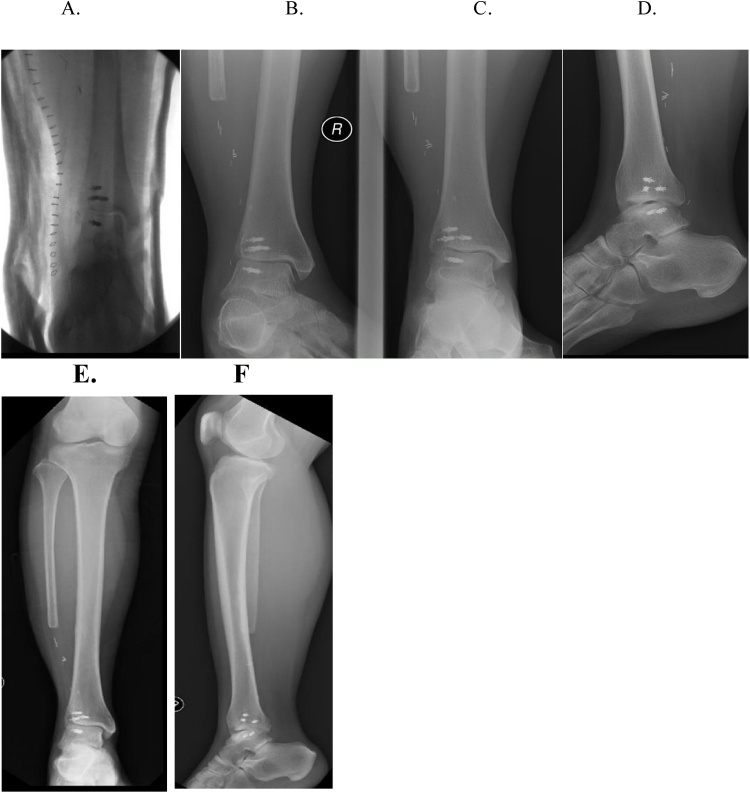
A) Immediate postoperative radiographs of patient #1 A, B, C, D) Two years post-op. E, F) Eight years post-op.
